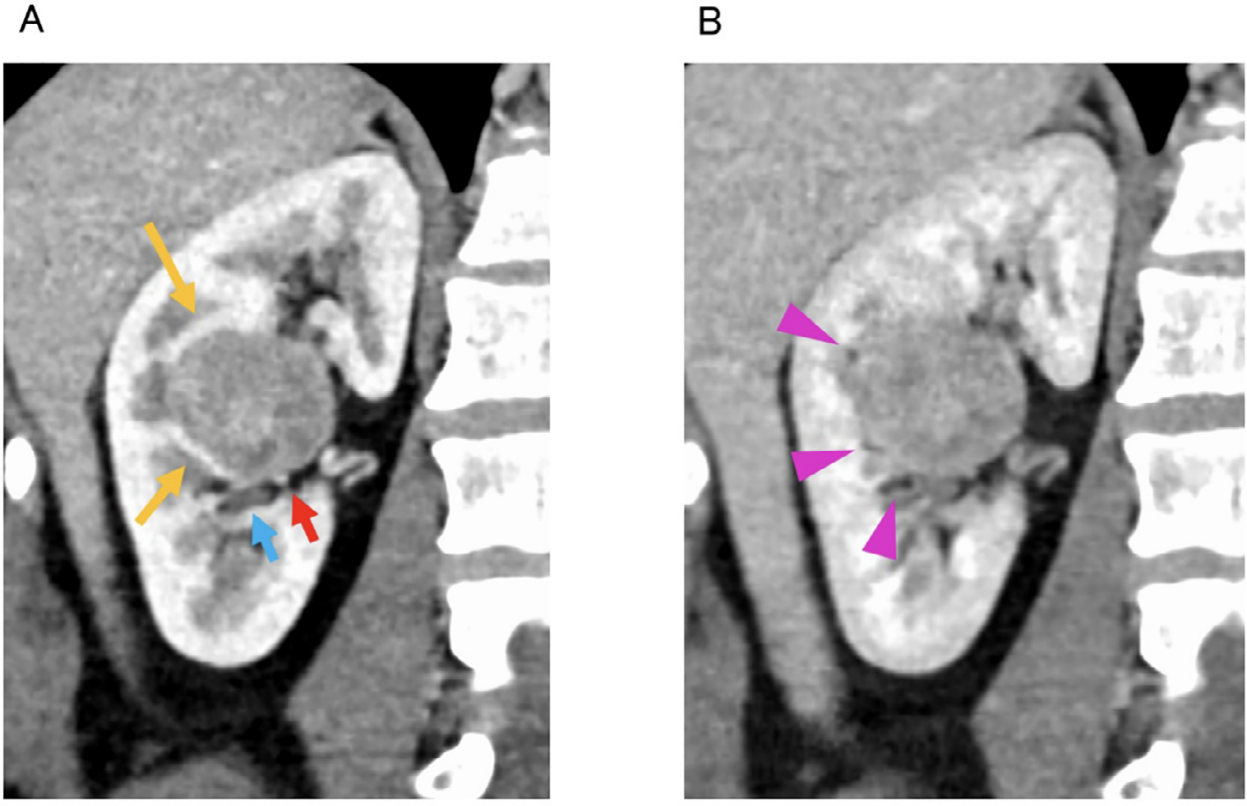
Coronal image of dynamic contrast-enhanced CT. (A) corticomedullary phase, and (B) nephrogenic phase. Tumor is compressing the renal parenchyma (yellow arrow). Vessels (red arrow), calyces (light blue arrow), and small fat (pink arrowhead) are present in multiple locations between the tumor and the renal parenchyma.
